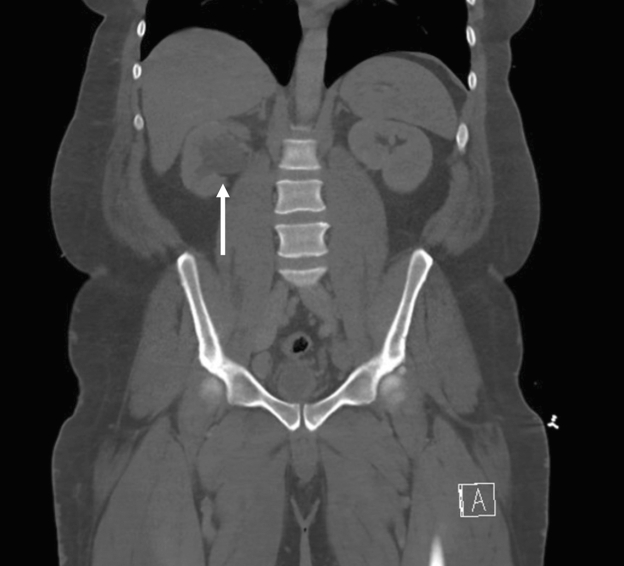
Computed tomography of the abdomen and pelvis demonstrating right hydronephrosis as denoted by the white arrow.
